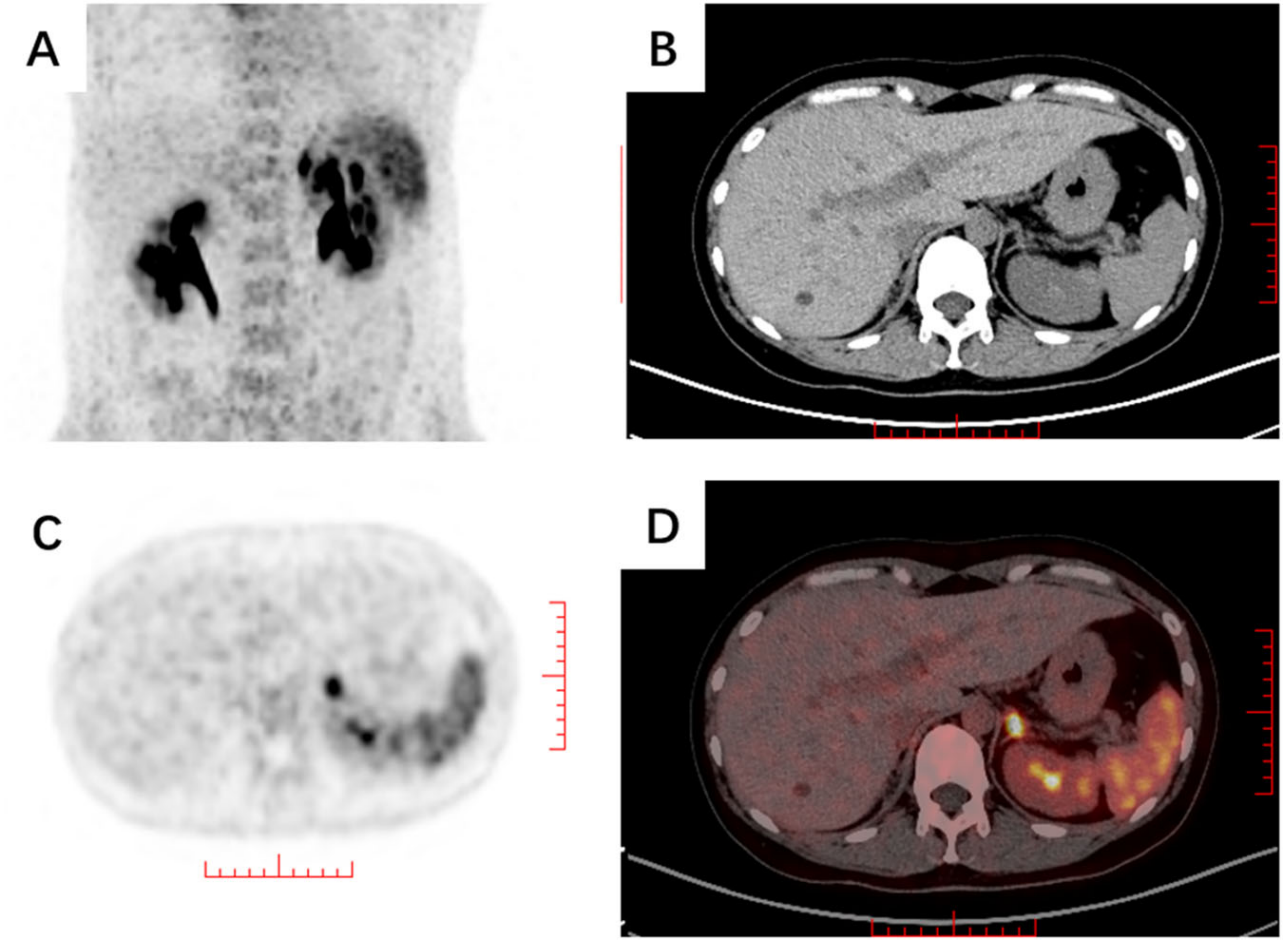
CXCR4-targeted PET/CT nuclear imaging results. A PET maximum intensity projection shows increased metabolic activity near the medial limb of the left adrenal body. B–D Unenhanced and contrast-enhanced CT and fused images display a uniform density left adrenal nodule with increased metabolism, SUVmax 8.75
